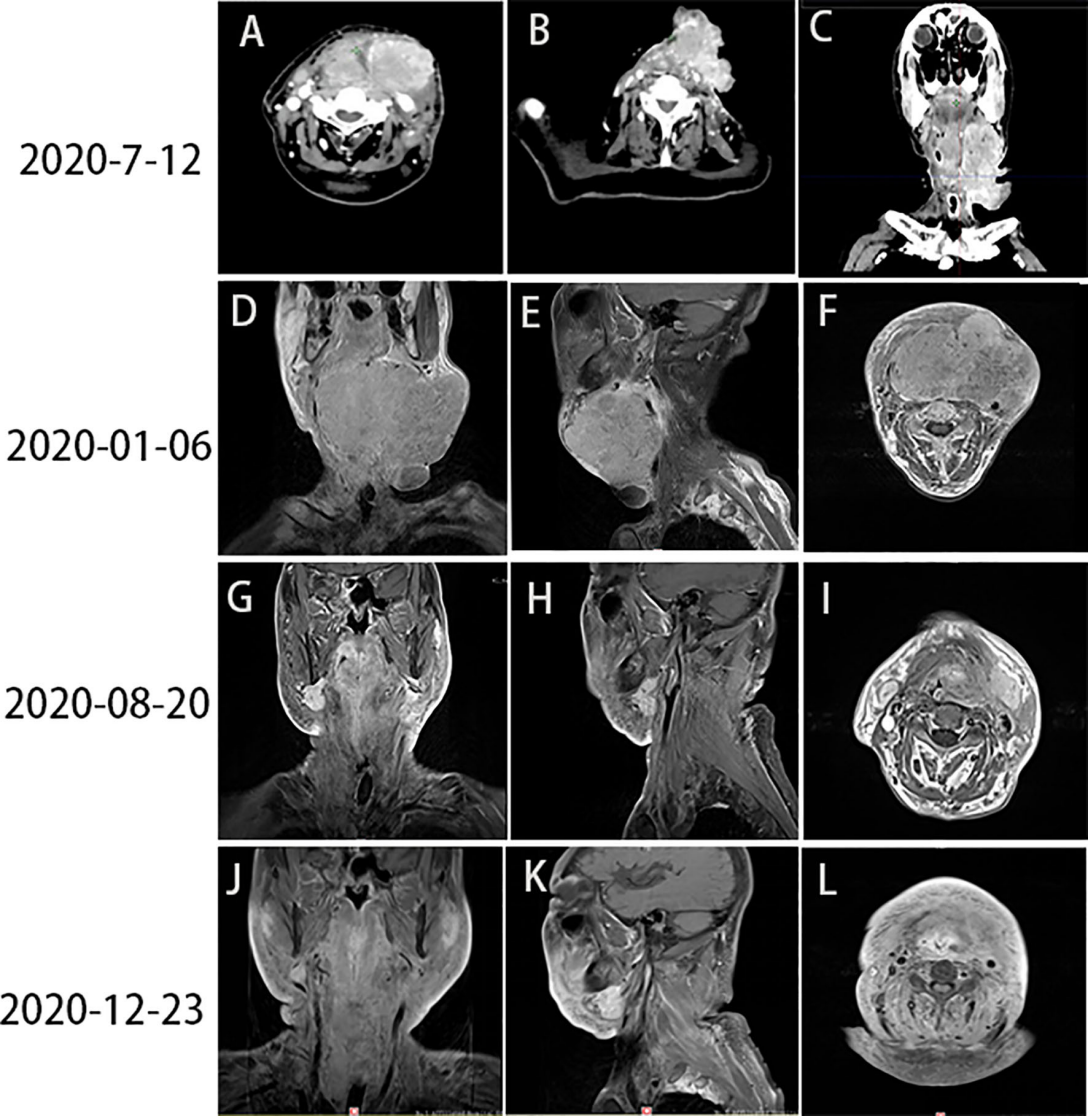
(A–C) Head and neck CT revealed PD (June 12, 2020). (D–F) Images captured prior to radiotherapy (January 6, 2020). (G–I) Images captured after the end of radiotherapy (August 20, 2020). (J–L) The tumor mass had almost completely regressed by December 23, 2020. CT, computed tomography; PD, progressive disease.
